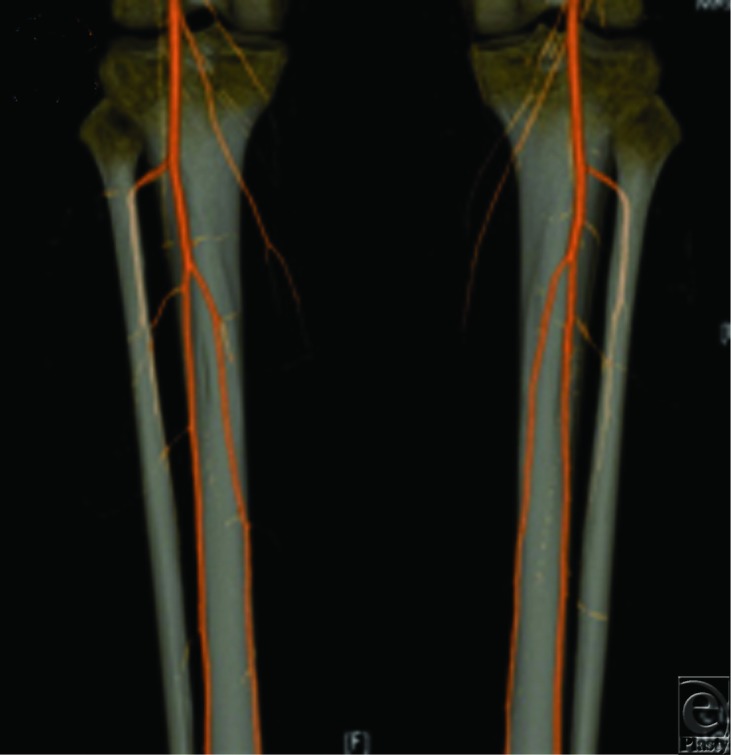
CTA of donor site for head and neck reconstruction. CTA of bilateral lower extremities in a patient with ameloblastoma of the mandible demonstrating bilateral peroneus magnus.
